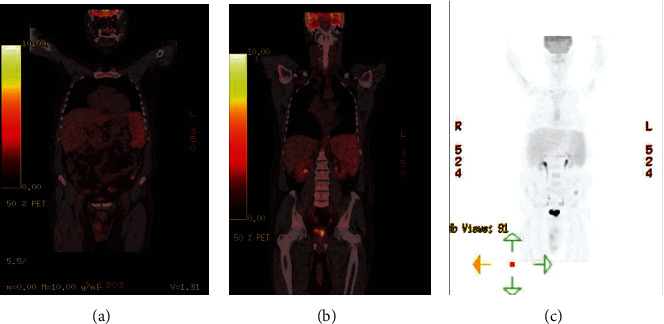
PET-CT scan. Initial PET-CT. (a) Shows the hypermetabolic lesions, which can be seen in the descending colon, and the augmented signaling of the liver without renal compromise is documented. (b, c) PET-CT scans showing nonenhancement of the kidney signal.
